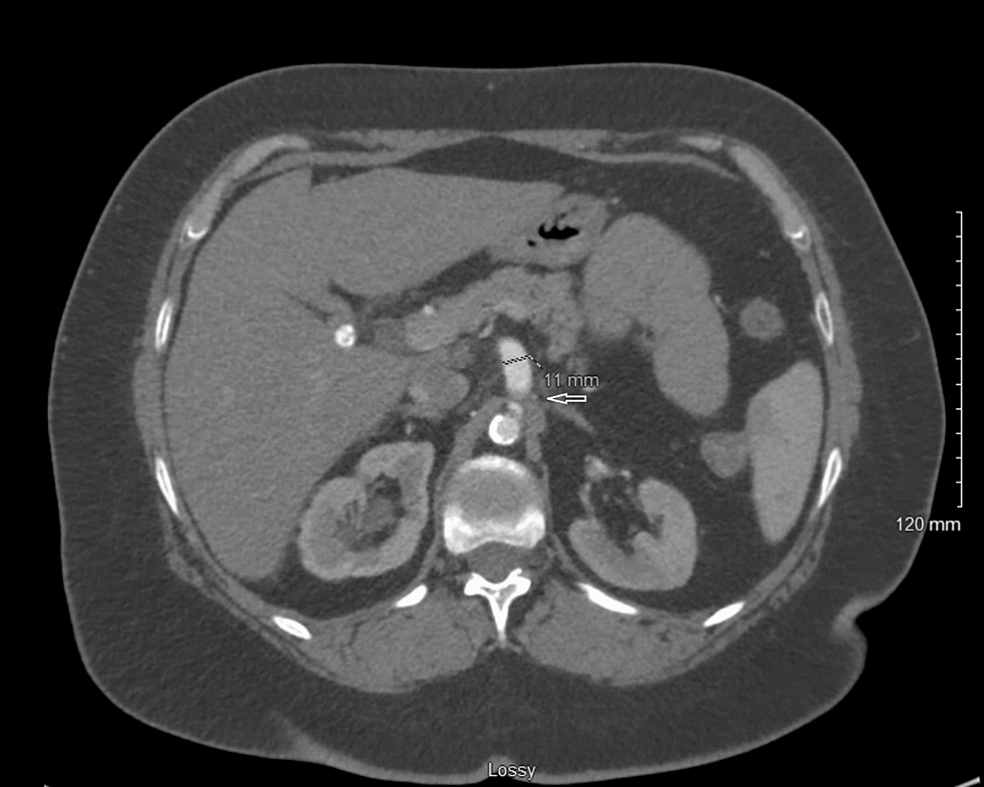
CT abdomen showing narrowed celiac artery
